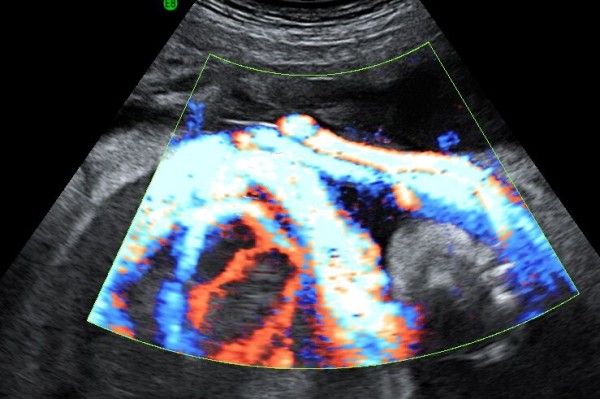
Color Doppler of giant placental choriangioma.
